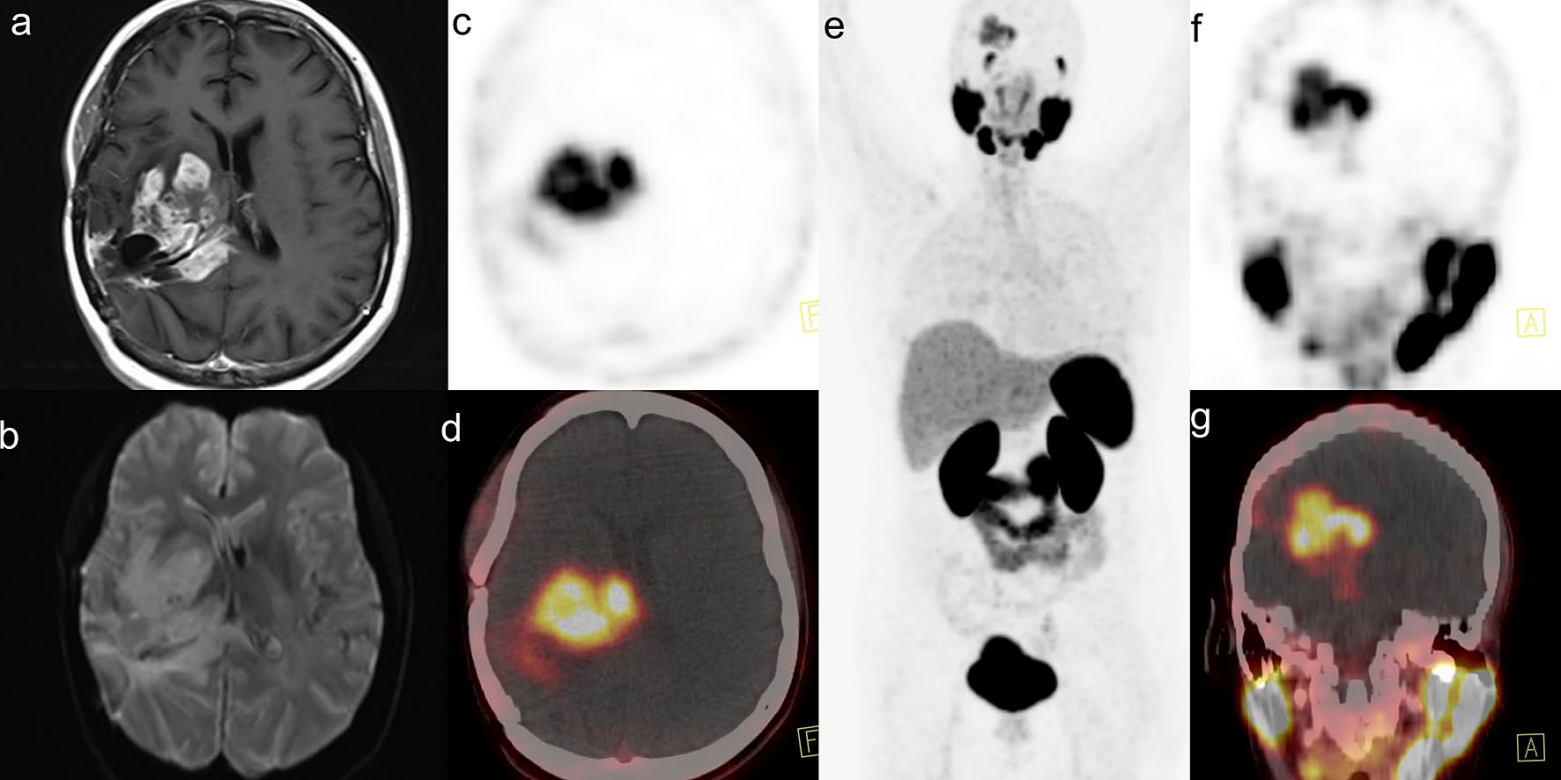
Male, 39 years, with a recurrent anaplastic astrocytoma IDH wildtype grade III in the right parieto-occipital lobe. Three years after surgery, and 15 months after local radionuclide therapy with [^213^Bi]Bi-DOTA-SP, a contrast-enhanced mass around the surgical cavity was detected with MR. [^68^Ga]Ga-PSMA-11 accumulation in the tumor was found with the SUV_max_ 4.9, SUV_mean_ 3.3, TBR 70 and TLR 0.8 (a—contrast-enhanced T1WI, b—DWI, c—PET transverse image, d—fused PET/CT transverse image, e—maximal intensity projection, f—PET sagittal image, g—fused PET/CT sagittal image).
